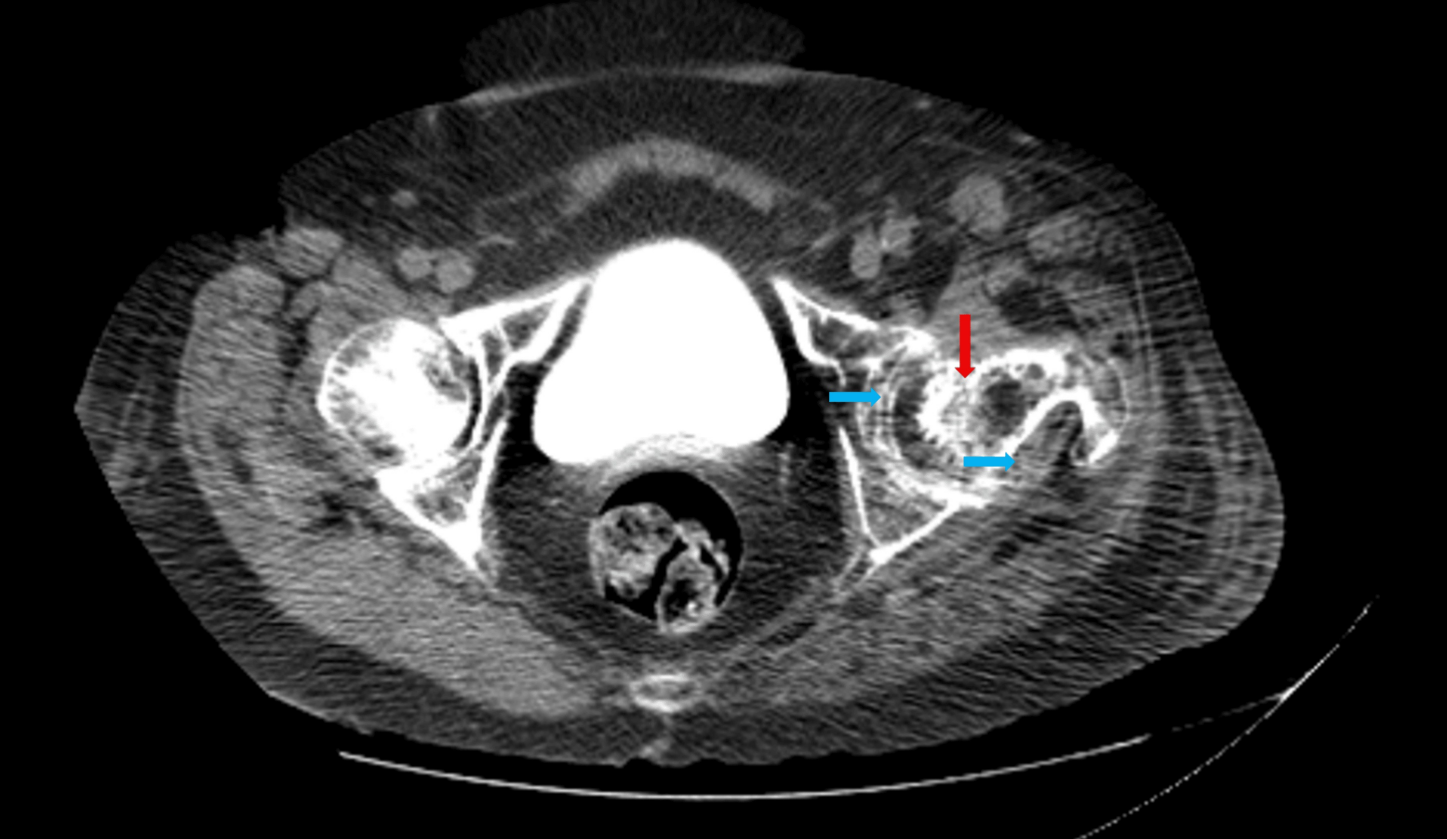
CT scan of the left hip. Extensive destruction and irregularity of the femoral head (vertical, red arrow), accompanied by joint effusion and periarticular soft tissue swelling (horizontal, blue arrows).
